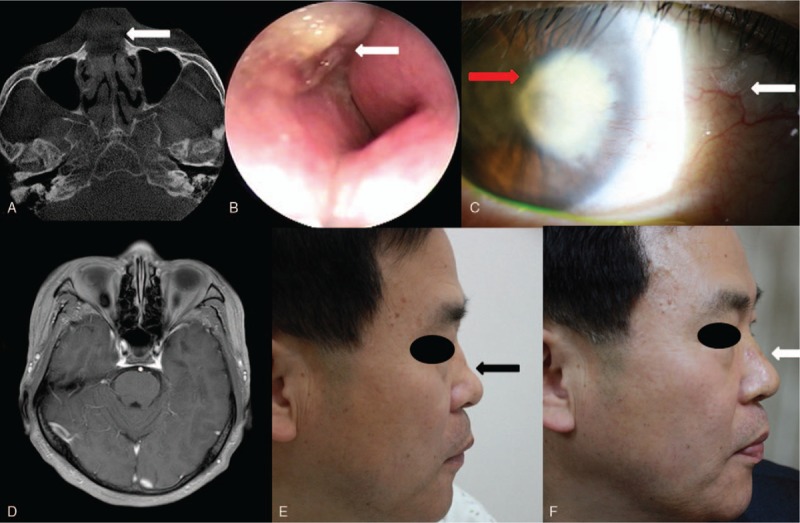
A. Computed tomography of the paranasal sinus. Enlarged septum with fluid content is indicated by a white arrow. Note the minimal air space in both nasal cavities. B. Nasal endoscopy of the left nasal cavity. Swelling of the septum can be seen (white arrow) despite previous incision and drainage. C. A slit-lamp examination revealed a fan-shaped whitish corneal opacity on the medial side of the right eye (red arrow). Neovascularization (white arrow) indicates a progressive lesion. D. Brain magnetic resonance imaging (MRI) showed a normal sigmoid sinus. E. A preoperative lateral photograph of the patient. Note the saddle nose caused by the septal abscess and septal necrosis (black arrow). F. A postoperative lateral photograph of the patient. Implanted costal cartilage now supports the saddle nose and necrotic septum (white arrow).
